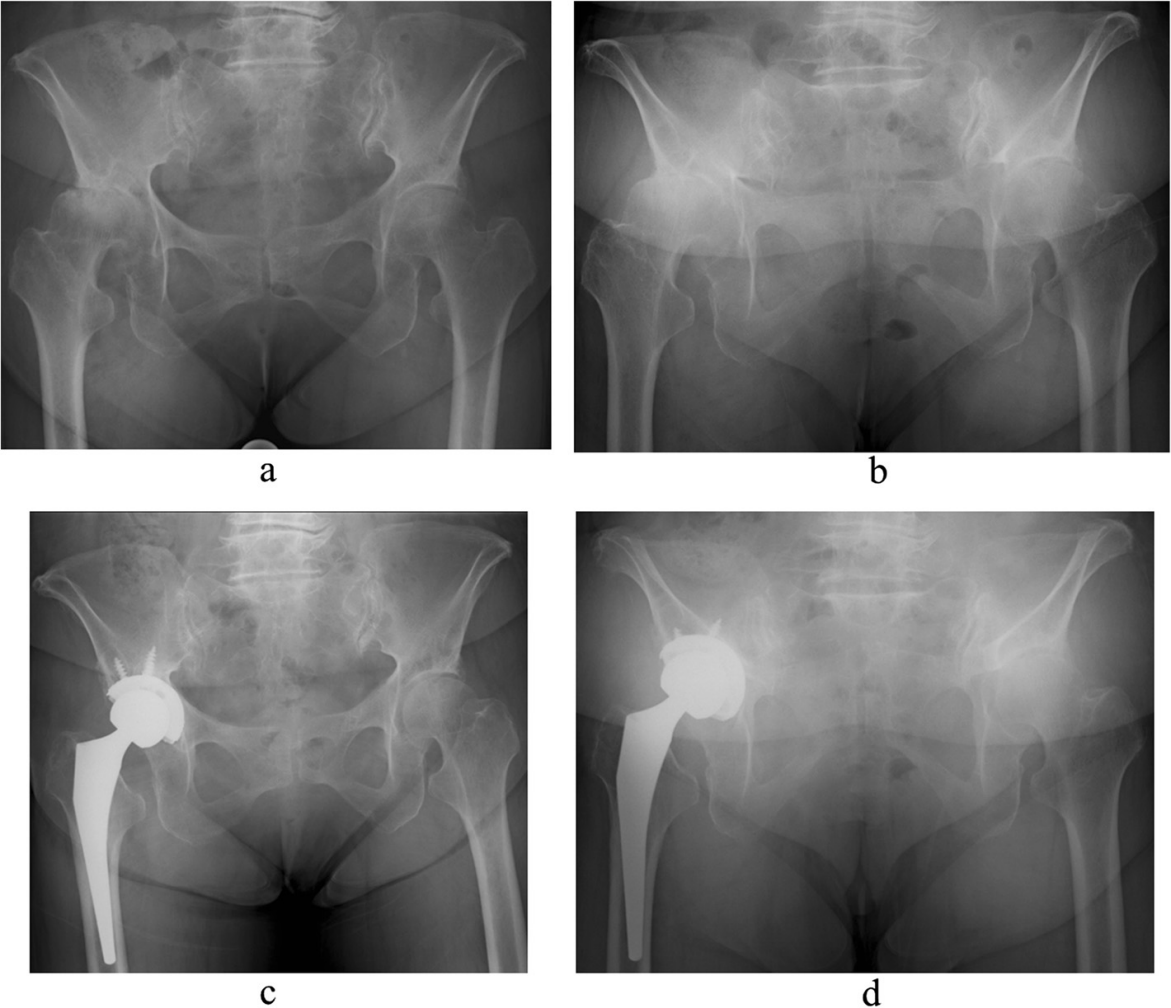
A 70-year-old woman with right hip osteoarthritis. On plain pelvic radiographs taken before right total hip arthroplasty (THA), the pelvis tilts backward by 18° in the supine position (a) and 34° in the standing position (b). Because the lumbolordotic angle had decreased to 5° in this case, the target reference plane for preoperative planning was set at retroversion of 30°. On radiographs taken at 1 year after THA, the pelvis tilts backward by 24° in the supine position (c) and 38° in the standing position (d), and combined anteversion (CA) is 31.3° in the supine position and 39.2° in the standing position. Because the ideal CA value of 37.3° is situated between the CA values in the supine and standing positions, the pelvic tilt is less likely to deviate from 37.3° even with further progression of posterior pelvic tilt in the future
